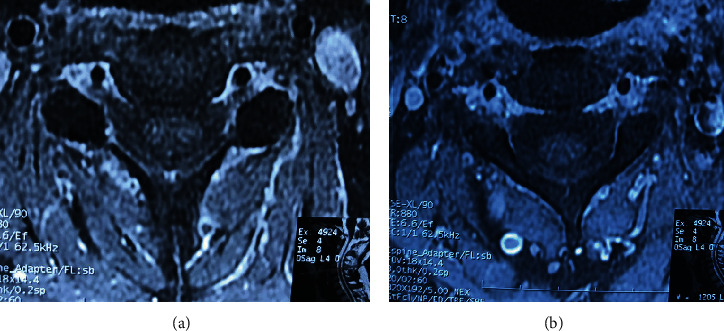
T1, axial MRI showing spinal canal stenosis.
